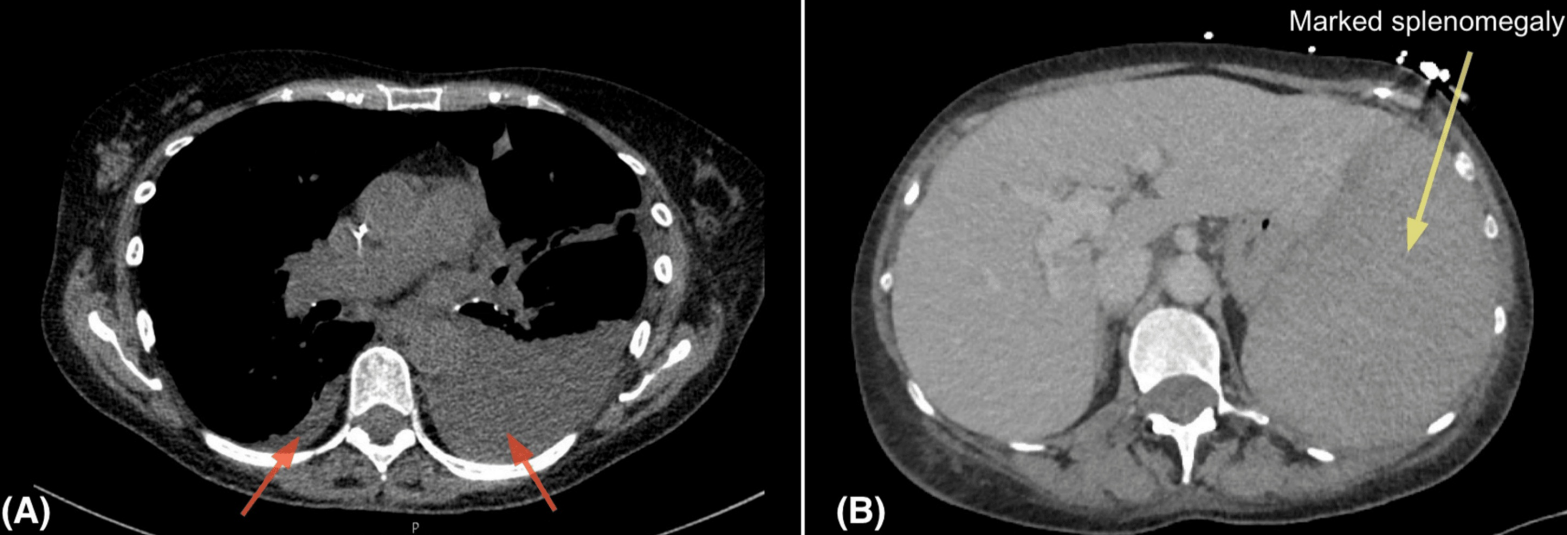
CT imaging demonstrating pleural effusions and splenomegaly (A) Axial high-resolution CT (HRCT) chest showing bilateral pleural effusions, larger on the left (red arrows). (B) Axial contrast-enhanced CT abdomen showing marked splenomegaly (yellow arrow).
